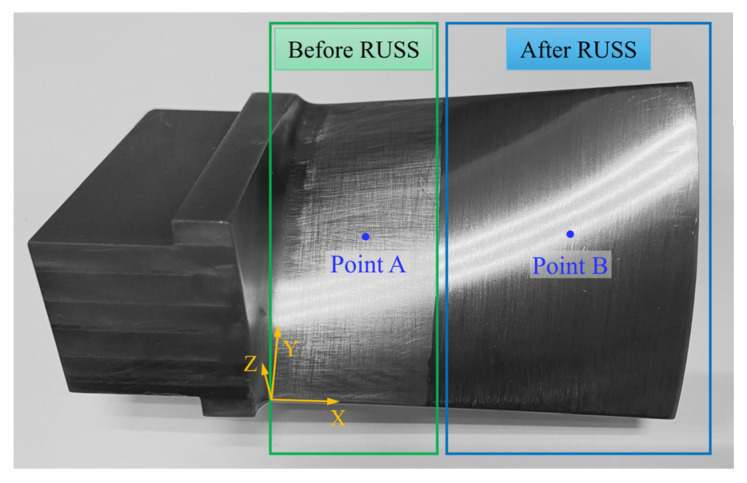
Aviation blade surface before and after robotic ultrasonic surface strengthening (RUSS).
